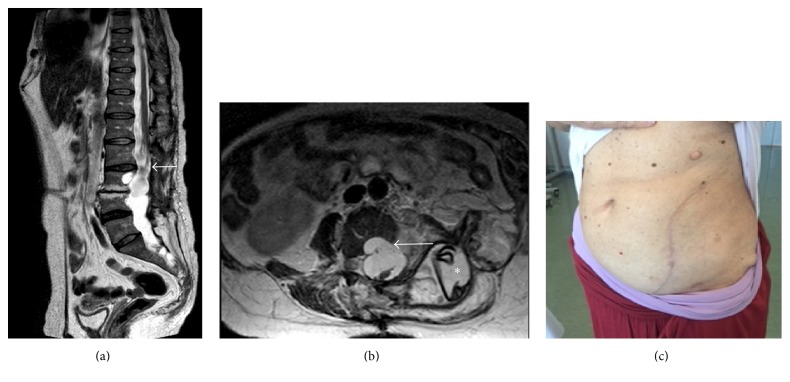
After six months, the patient was fine without neurological deficit or abdominal symptoms and the spinal sagittal and axial T2-weighted MRI images showed no relapse of the pseudomeningocele (a and b). The sagittal T2-weighted MRI demonstrated the decompression of cauda roots (white arrows), even if appeared clumped together due to postsurgical scar. In the axial T2-weighted MRI the residual meningocele (white arrow) and the ADM (asterisk) with fat patch were demonstrated. The surgical incision completely healed was shown in image (c).
